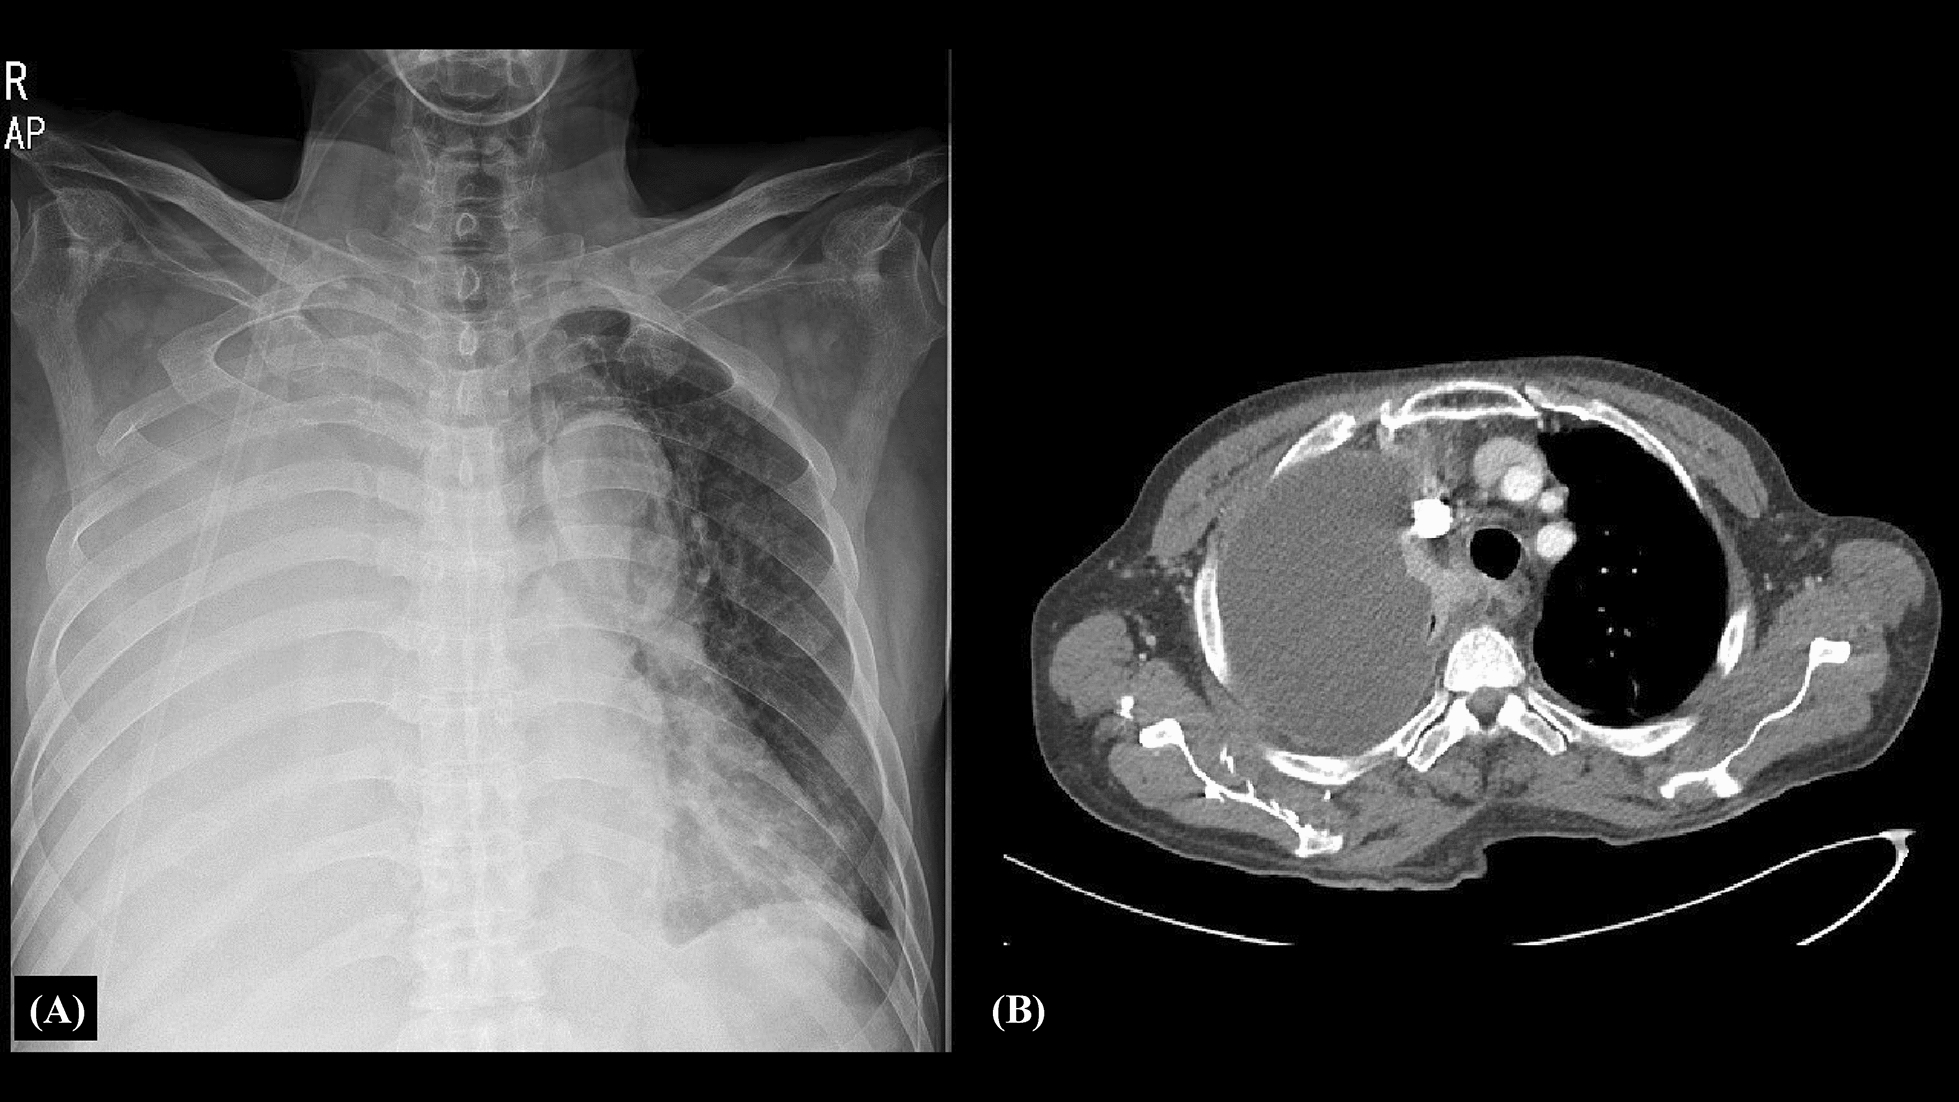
A, B Chest radiograph and chest computed tomography. The chest radiography showed a totally whited-out right lung and chest computed tomography revealed a large amount of right pleural effusion and diffuse pleural enhancement
